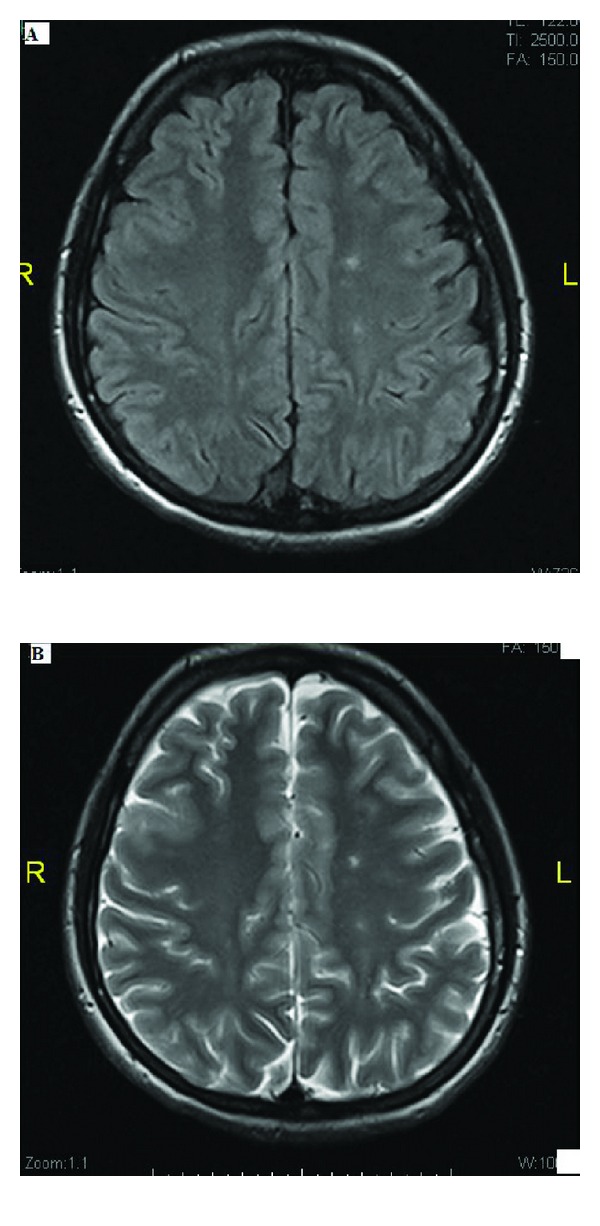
Brain magnetic resonance image (MRI) shows left periventricular white matter hyperintensities in FLAIR image (A), and left periventricular white matter hyperintensities in axial T2-weighted brain MRI (B).
